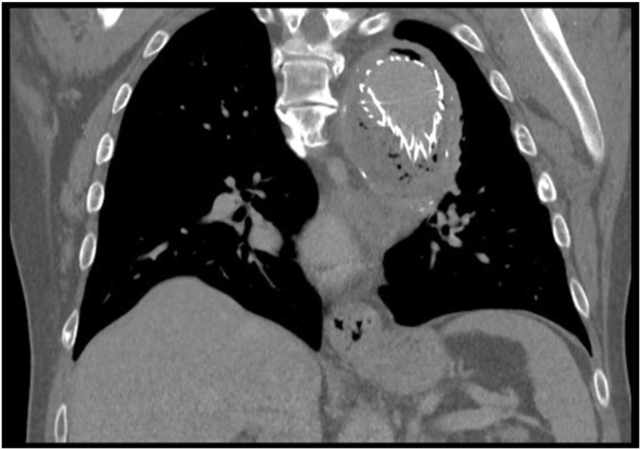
Computed tomography of the infected aneurysm sac and stent graft at the time of diagnosis.
